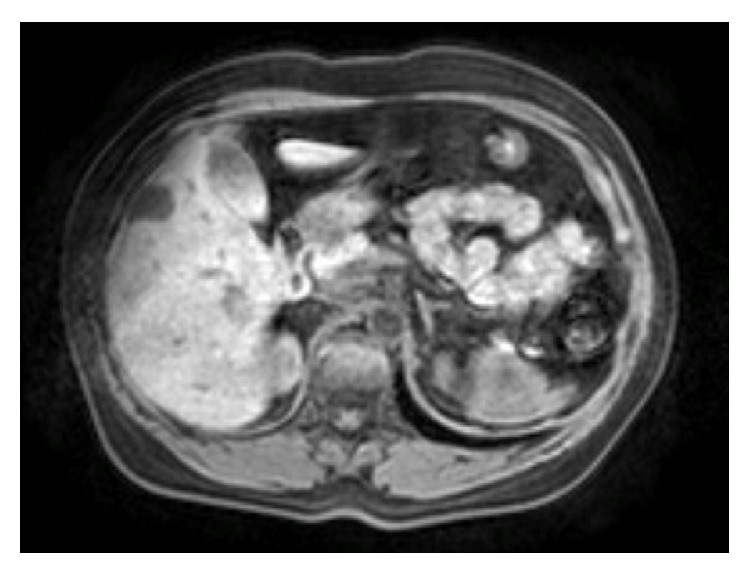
MRI demonstrating solitary segment V liver metastasis.
